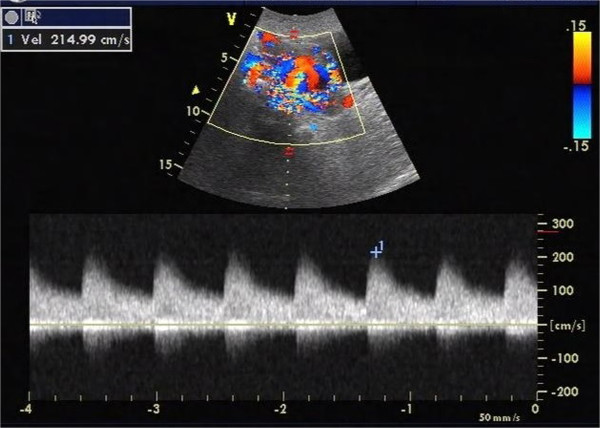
Blood flow velocity within the supplying artery. Pulsed Doppler ultrasonography showed that the velocity of blood in the supplying artery near the orifice of the pseudoaneurysm was as high as 215 cm/s.
